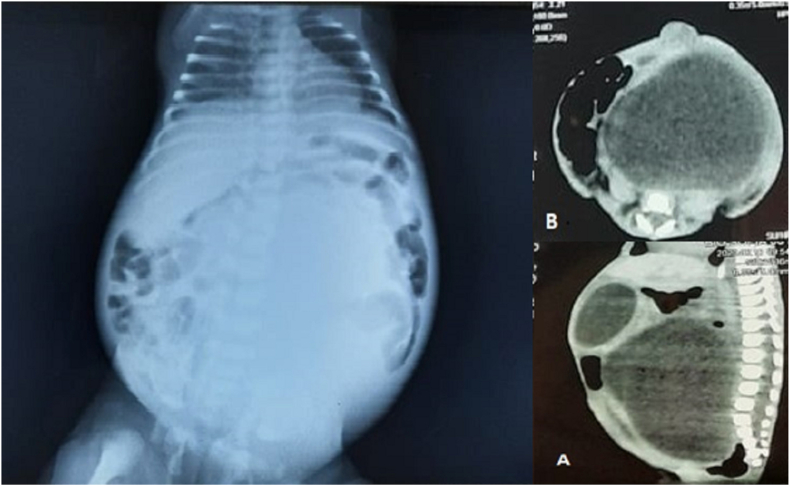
X-ray abdomen and computed tomography showing hydrometrocolpos compressing against the diaphragm causing respiratory distress.
